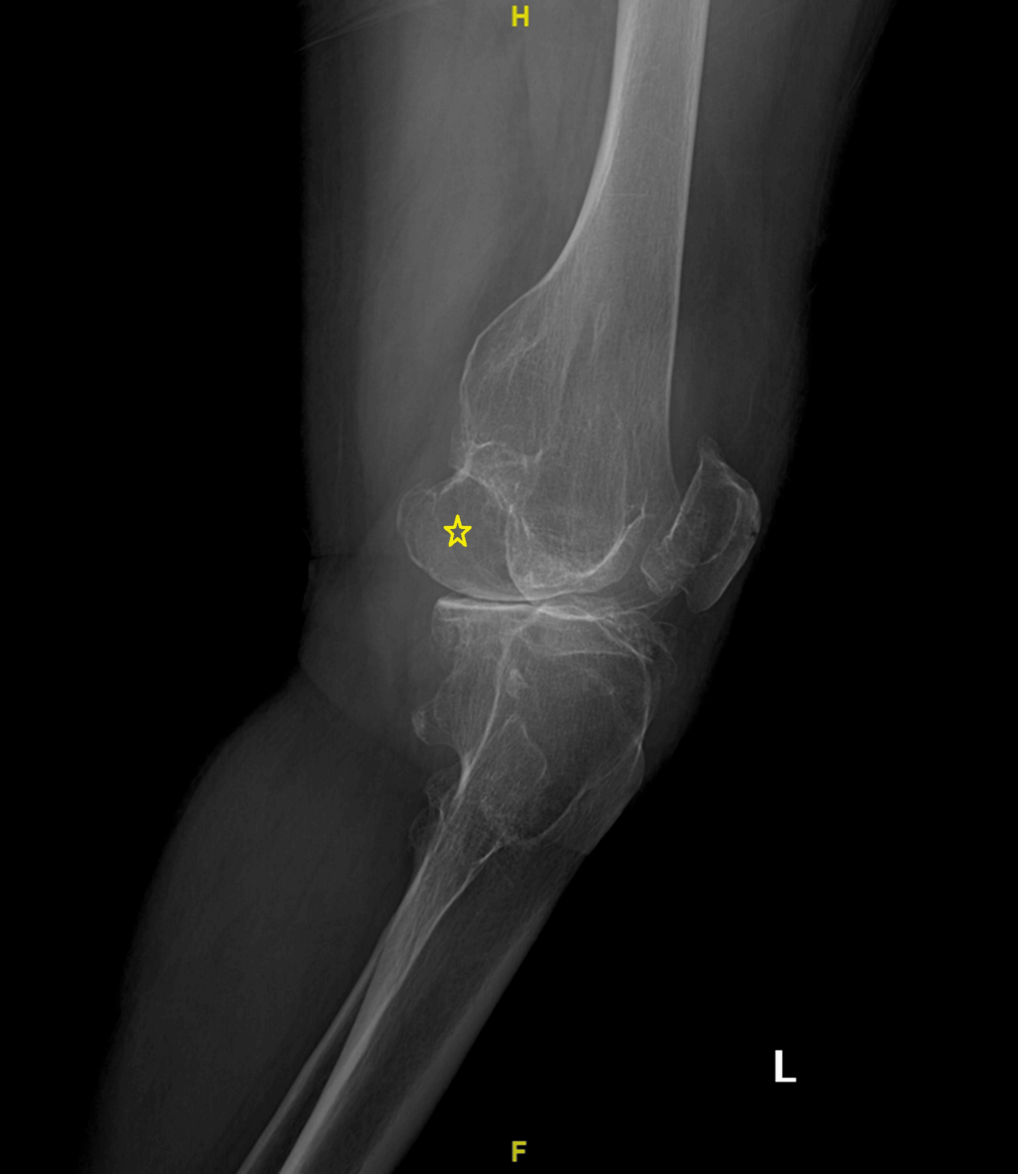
X-ray of the left knee in December 2015, showing the large exostosis.
